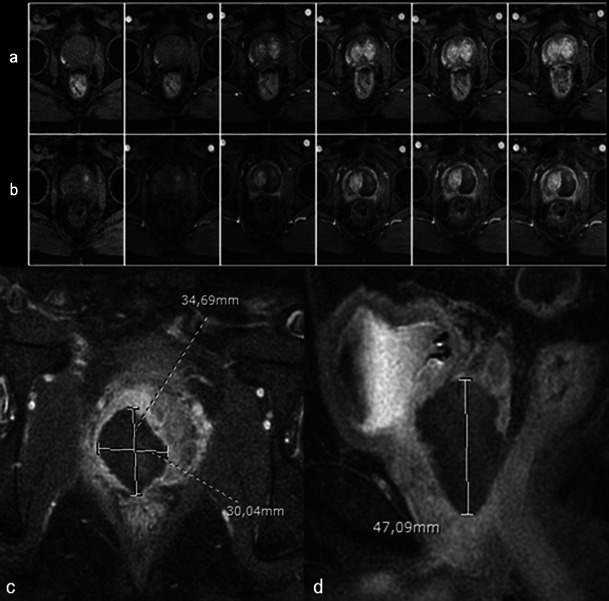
Well-defined necrosis boundaries. A 72-year-old patient with a PSA level of 4.5 ng/ml treated for Gleason 6 (3 + 3) left lobe adenocarcinoma. Axial dynamic contrast-enhanced images before treatment (a) show a zone of post-biopsy haemorrhage on the right lobe without any suspicious enhancement. Day-7 post-treatment left MRI (b) shows no enhancement of the left treated lobe (necrosis). Note the extended periprostatic enhancement that surrounds the whole gland and indicates inflammation. Right lobe treatment for Gleason 6 adenocarcinoma: day-7 post-treatment axial (c) and sagittal (d) FS contrast-enhanced images show a large homogenous hypointense non-enhanced area in the treated lobe (necrosis) with well-defined and highly enhanced boundaries
